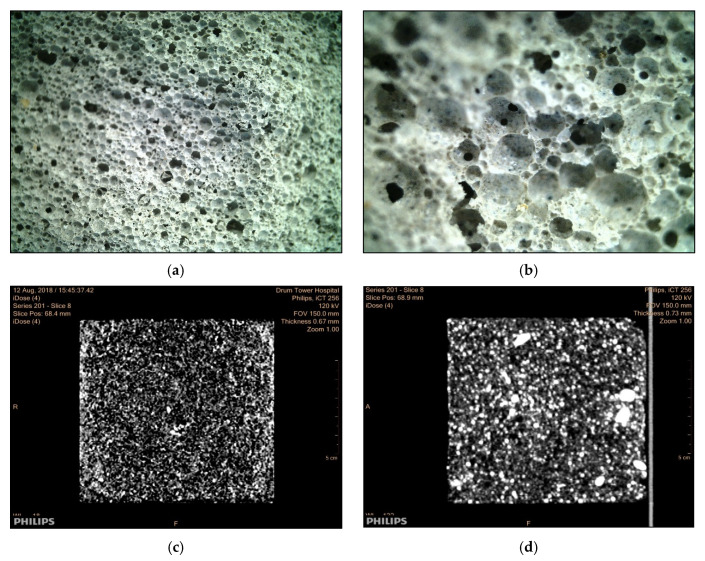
Pore morphology of the foam backfill material with different *W/B* ratios. (a) 0.40 *W/B* ratio, (b) 0.50 *W/B* ratio, (c) 0.40 *W/B* ratio through the CT scan, (d) 0.50 *W/B* ratio through the CT scan.
